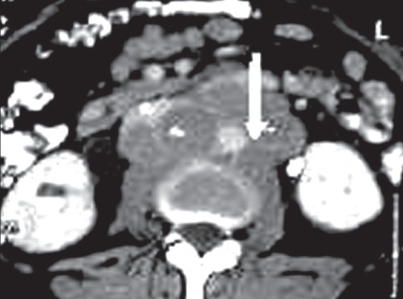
CT scan showing a para-aortic lymph nodal mass (arrow)
